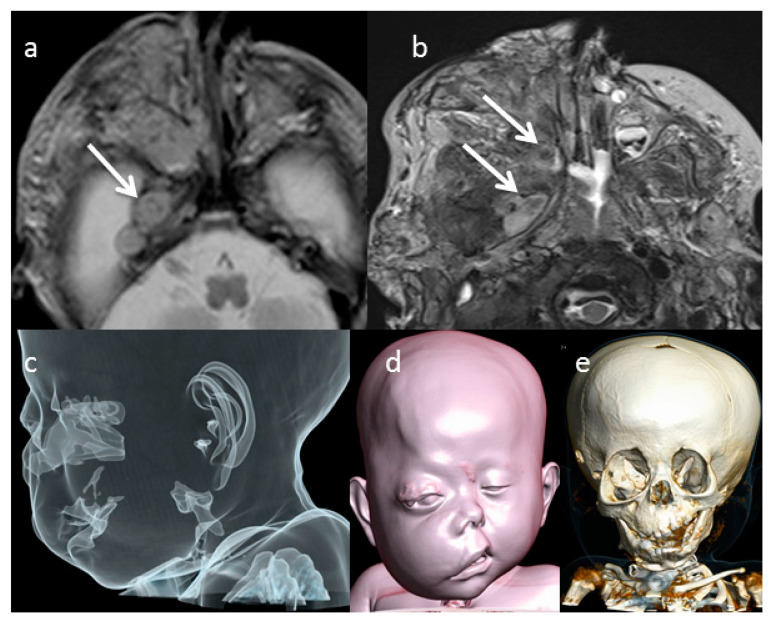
Axial T2-w MRI performed at diagnosis (a) and at the follow-up (b) showed the typical “target sign” represented by a rim of high signal peripherally and low signal centrally ((a,b) white arrows). Computed tomography (CT) 3D reconstructions (c–e) showed the swelling of the cheek and the ocular proptosis of the right eye (d) and the orbital enlargement (e).
